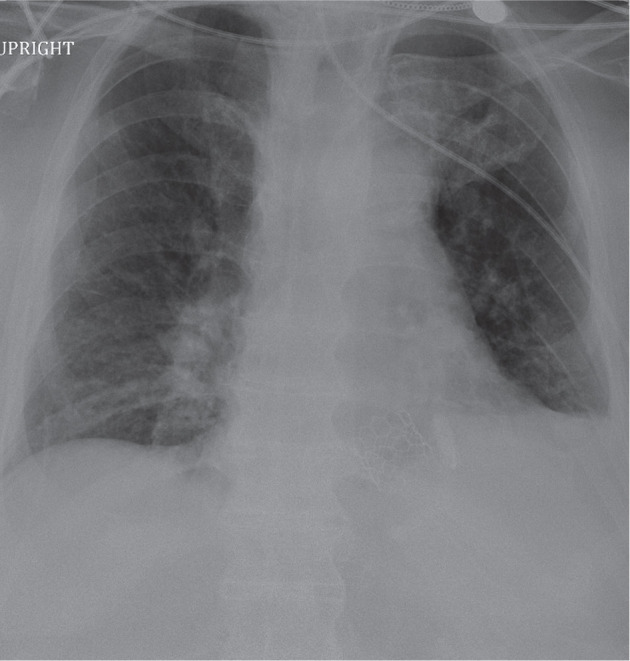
Chest radiograph showing the vertical orientation of the Micra™ upside down in the right ventricular outflow tract.
